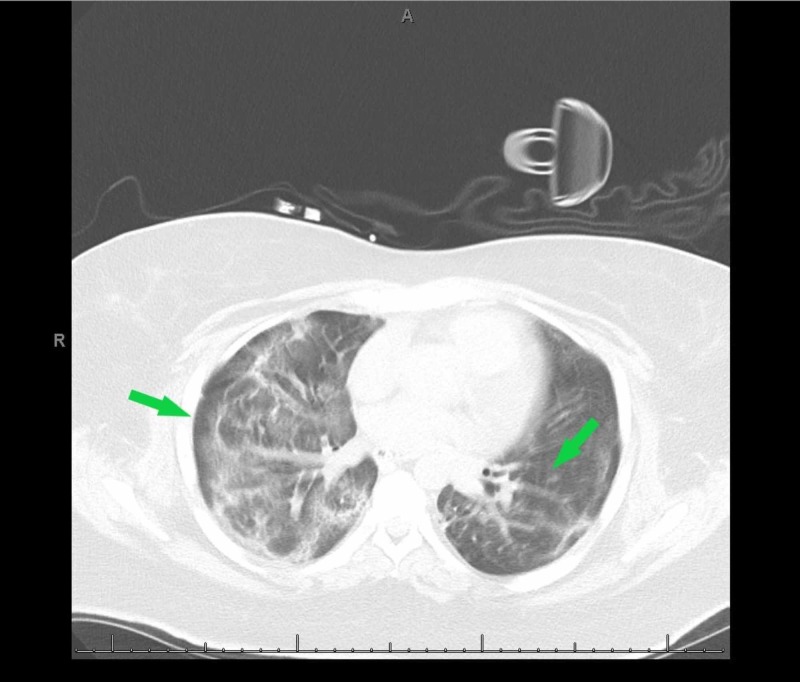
A computed tomography (CT) of the chest revealing long opacities in bilateral lungs with superimposed intralobular and interlobular septal thickening (arrows) consistent with acute respiratory distress syndrome (ARDS)
